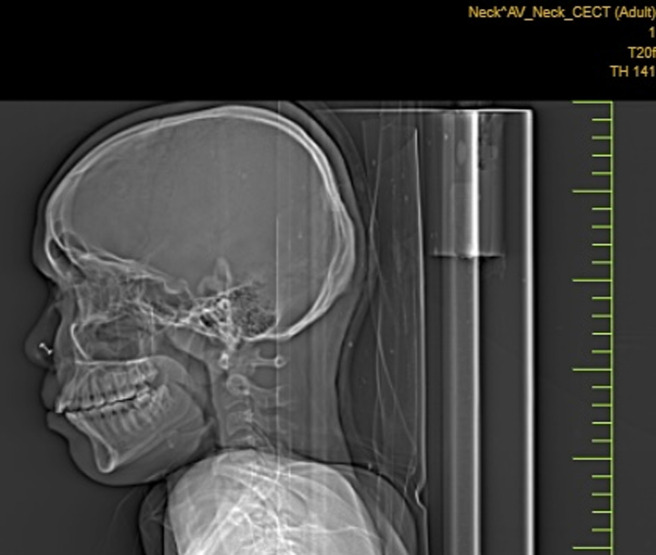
contrast enhanced computed tomography- cystic formation at the midline of the neck
